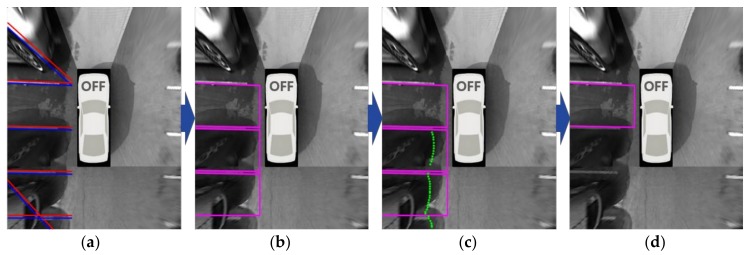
Parking slot candidate generation and occupancy classification. (a) Separating line detection results; (b) Parking slot candidate generation results; (c) Range data obtained by an ultrasonic sensor; (d) Parking slot candidates classified as vacant.
